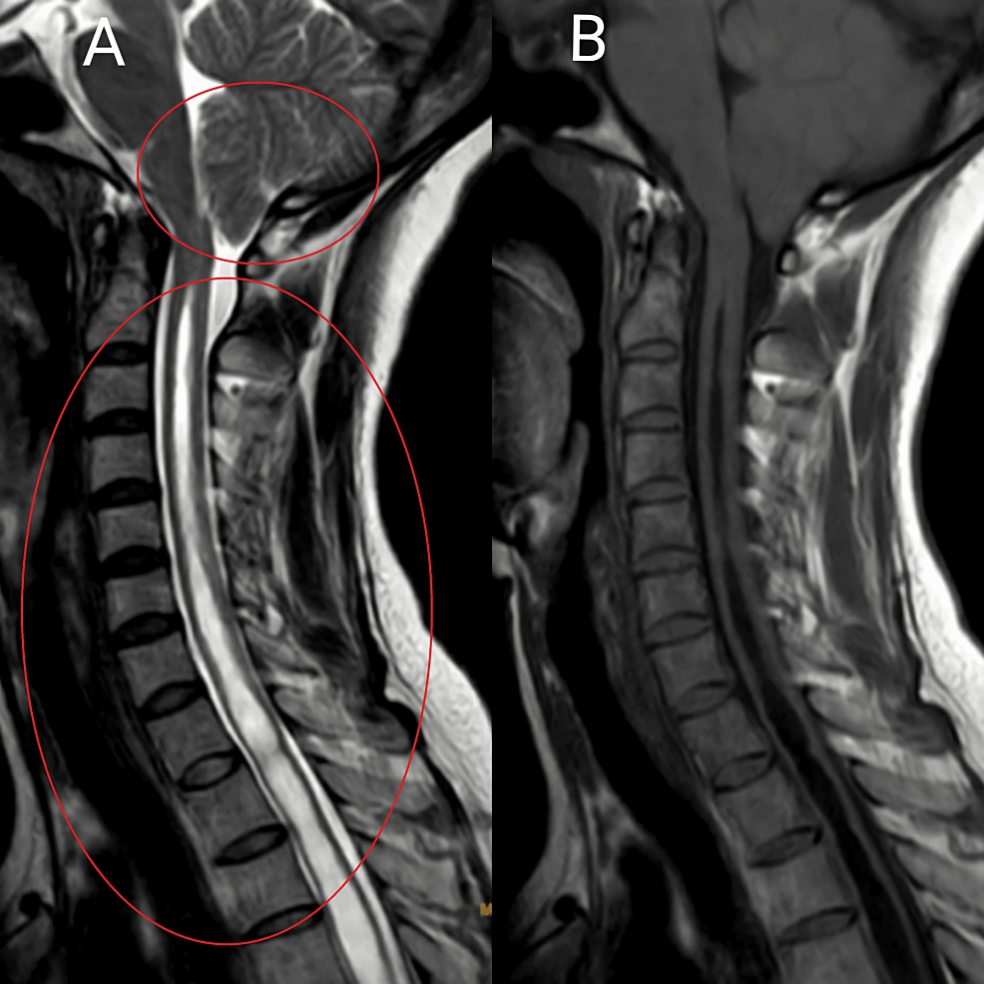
MRI of the cervical spine shows Arnold Chiari I malformation and syrinx formation from C2- D10 vertebral levels.
